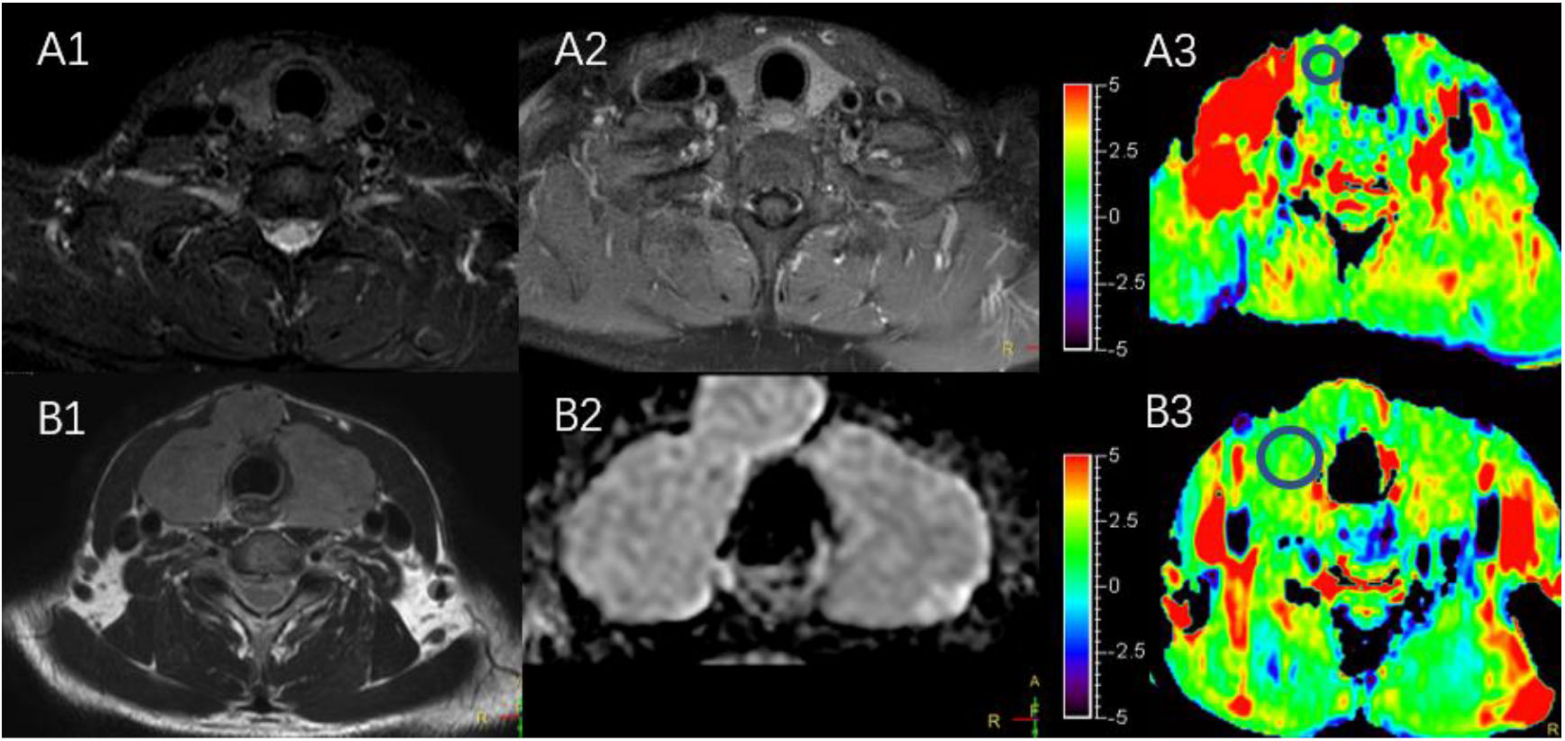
(A1–A3) MR images of a normal thyroid, including T2WI (A1), Gd-T1WI (A2), and APTWI (A3). (B1–B3) MR images of diffuse goiter, including T2WI (B1), ADC map (B2), and APTWI (B3).
